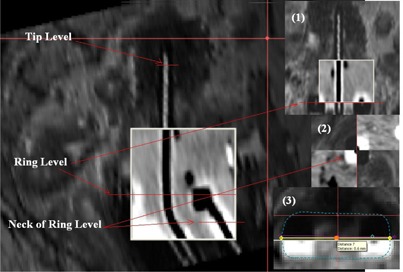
Verification of CT and MR image fusion of tandem and ring applicators on parasagittal plane with the positions of three verification sites. The inset 1, 2, 3 show the verifications of tandem and ring on paracoronal planes using dummy source, neck of ring on para‐axial plane, and the projected contours of ring applicators, reconstructed on CT and MR images, respectively.
